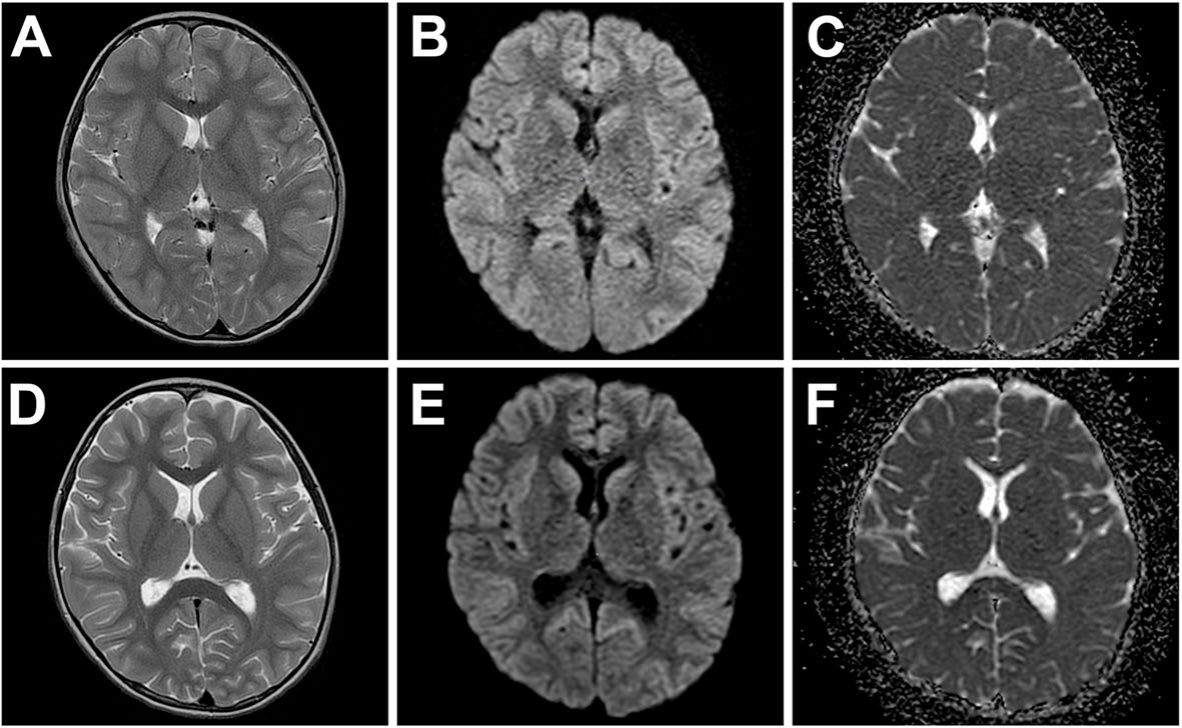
Brain MRI findings of the patient. Brain MRI images taken on the second (A, B, C) and eighth (D, E, F) days, where T2-weighted imaging (A, D), DWI (B, E), and ADC map (C, F). D, E, F Brain MRI on the eighth day shows no abnormalities except for mild cerebral atrophy
